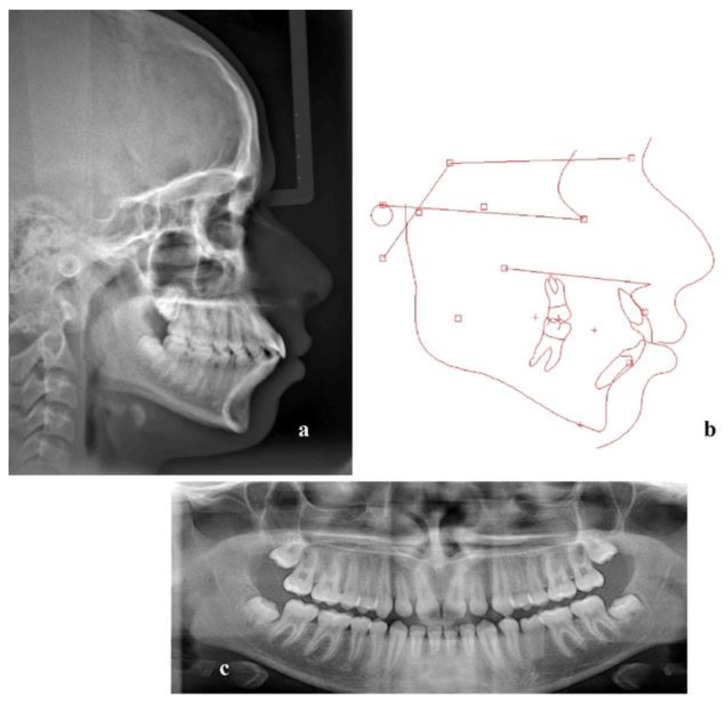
(a) Lateral cephalogram, (b) tracing and (c) panoramic X-ray before treatment.
